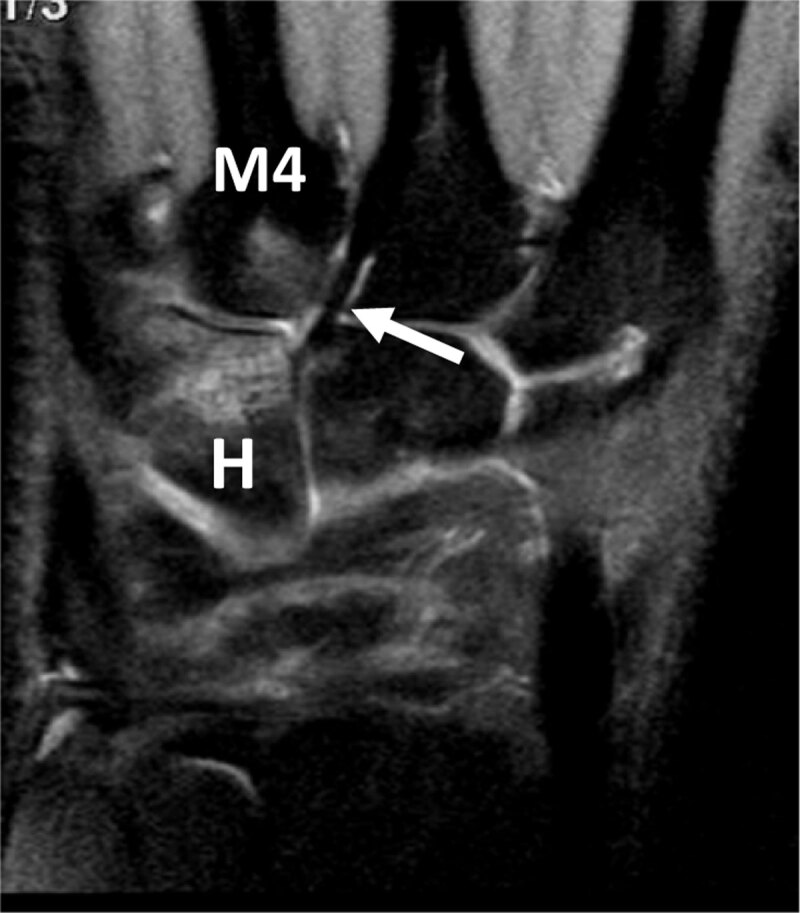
Hamate BME and associated BME of the fourth metacarpal base in an overused pianist hand. Coronal proton-density fat-saturated MR image depicts high signal bone marrow infiltration of the distal half of the hamatum (H) and proximal base of the fourth metacarpal (M4) nearby the capito-third metacarpal ligament (arrow) which is strong in this professional piano player.
